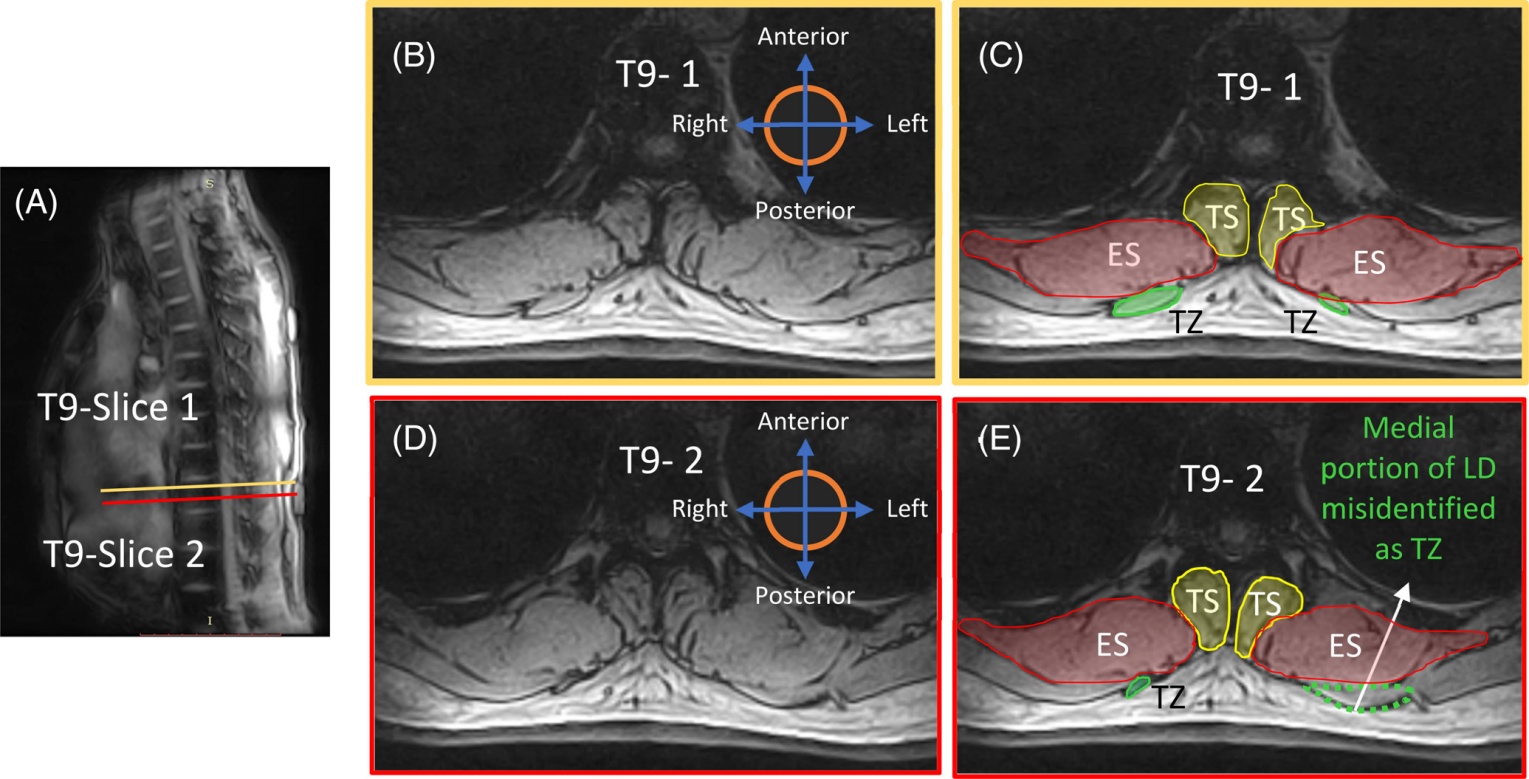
A, Sagittal MRI image showing the slice position and orientation at level T9. B, Transverse MR image of Slice‐1 at level T9. C, Segmentation of the correct ROI for TZ on Slice‐1. D, Transverse MR image of Slice‐2 at level T9. E, Misidentification of LD as ROI of TZ on MR image of Slice‐2 on T9. ES, erector spinae; LD, latissimus dorsi; ROI, region of interest; TS, transversospinalis; TZ, trapezius
